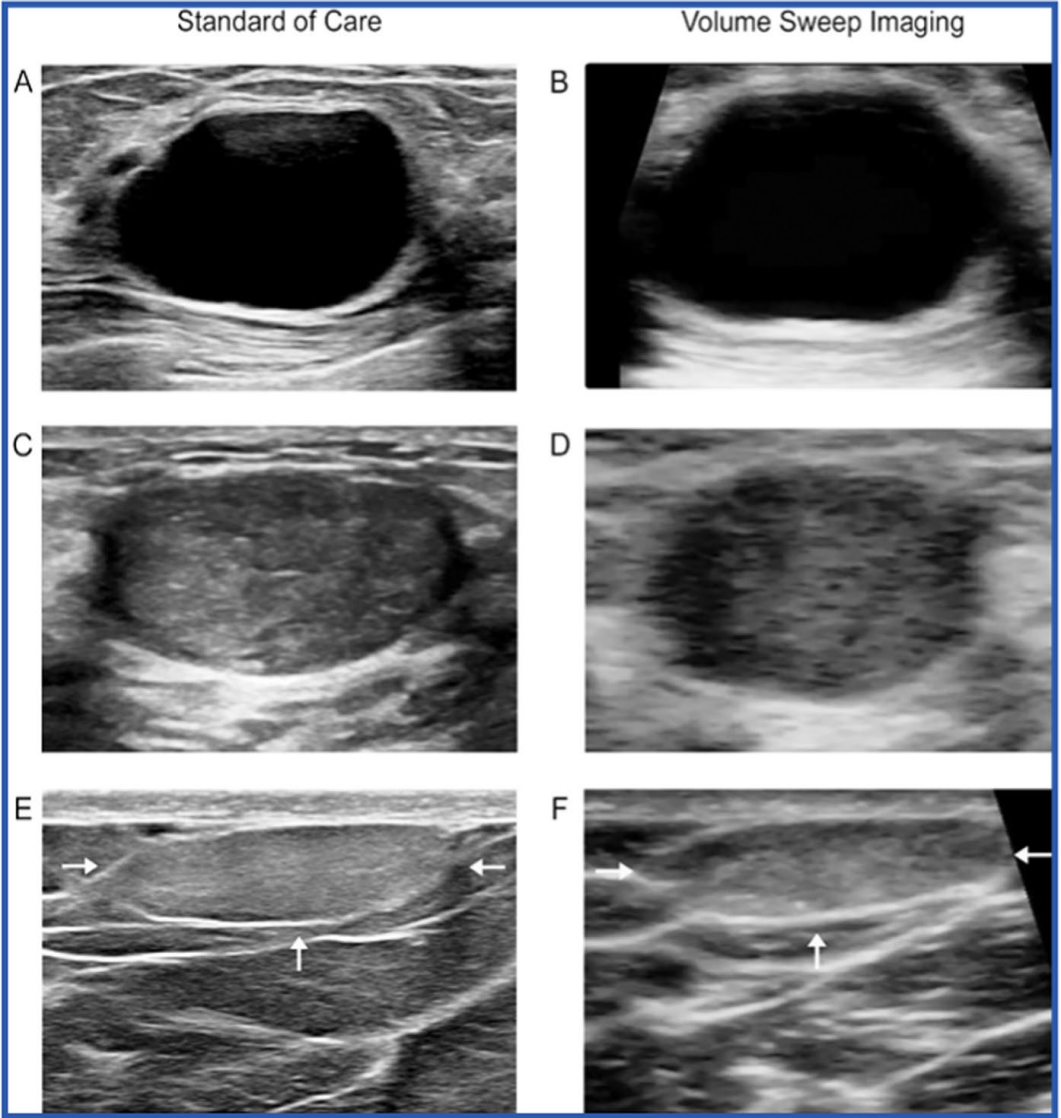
Benign breast lumps on standard of care ultrasound and volume sweep imaging (VSI). Benign anechoic cyst with posterior acoustic enhancement seen on standard of care ultrasound (A) and VSI (B). Hypoechoic well-circumscribed oval fibroadenoma seen on standard of care ultrasound (C) and VSI (D). Isoechoic or hyperechoic lipoma (arrows) in the superficial breast tissue on standard of care ultrasound (E) and VSI (F) [92]
